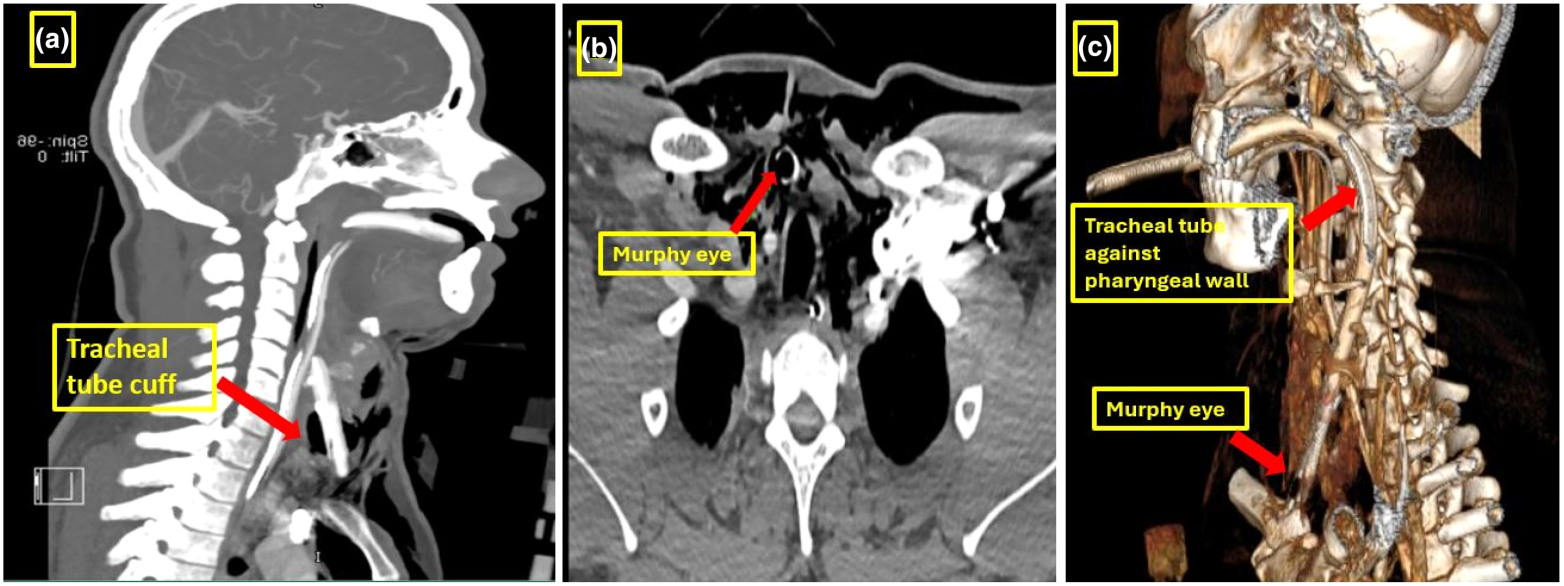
Computed tomography images showing (a) passage of the distal end of the tracheal tube into the anterior neck with inflated cuff and significant emphysema (b) location of the Murphy eye outside the tracheal lumen (c) a tracheal tube taking a curved course and lying against the posterior wall of the pharynx when known to be at a depth of 26 cm.
